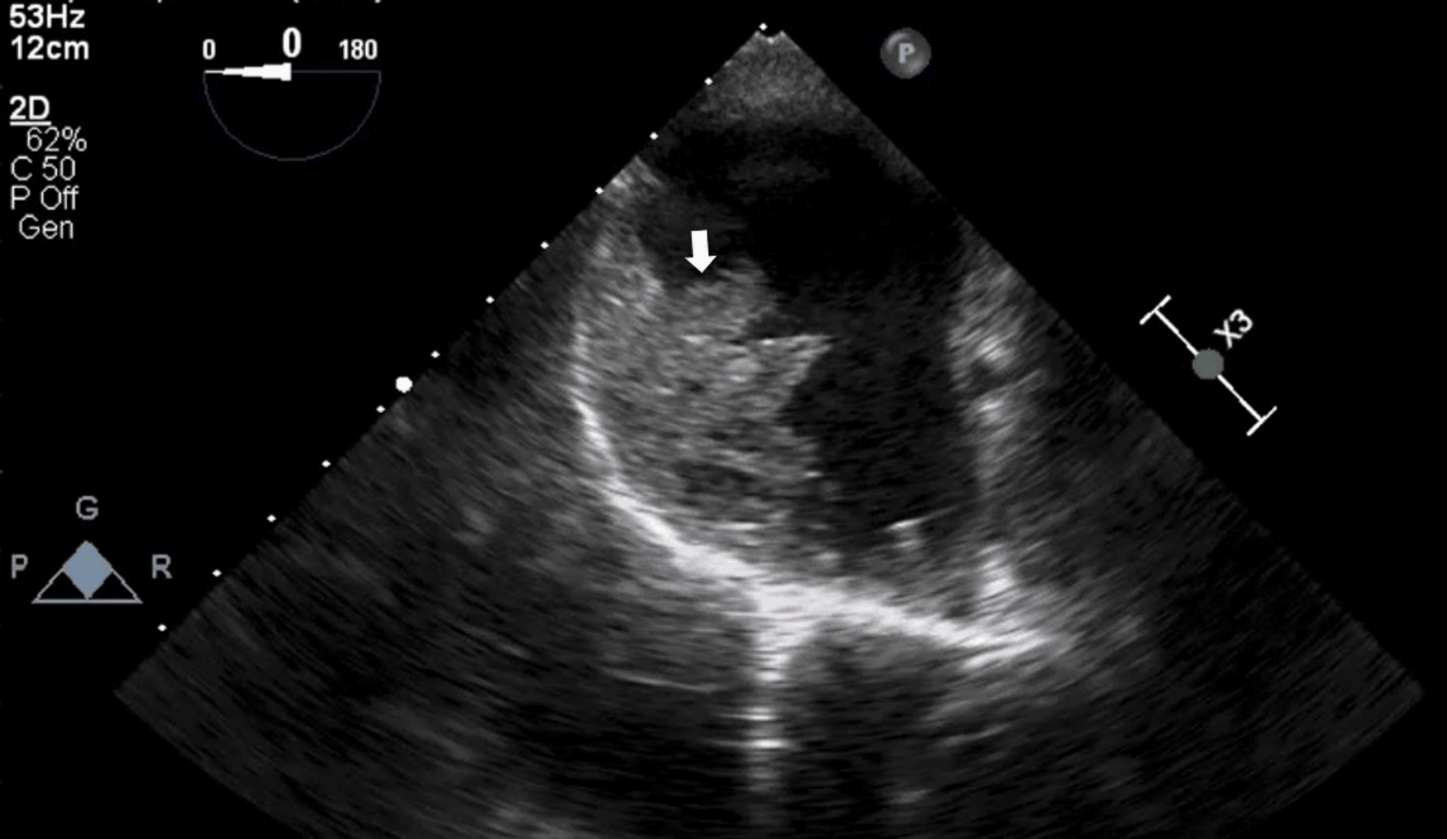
Transesophageal echocardiography (ECHO) showing a large right atrial thrombus (white arrow) measuring 8.7 × 12.1 cm.
